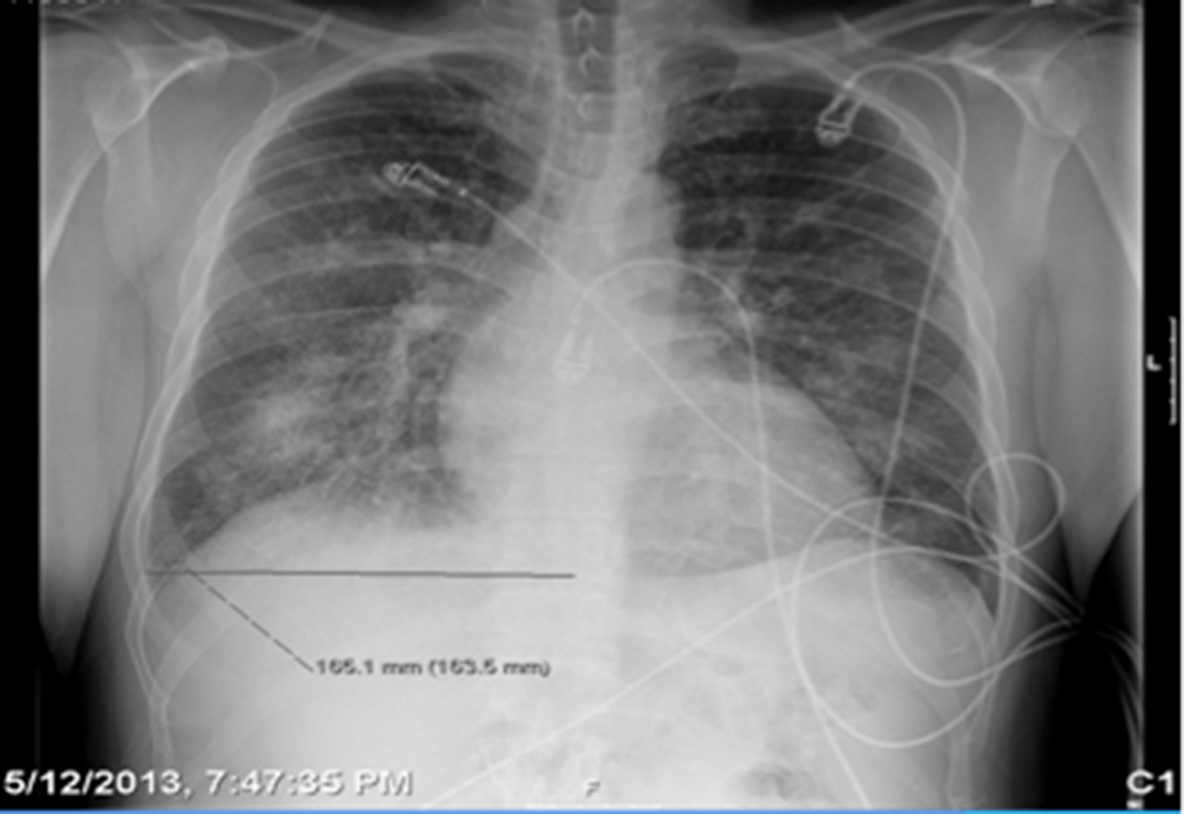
Chest X-ray- bilateral patchy opacities.
